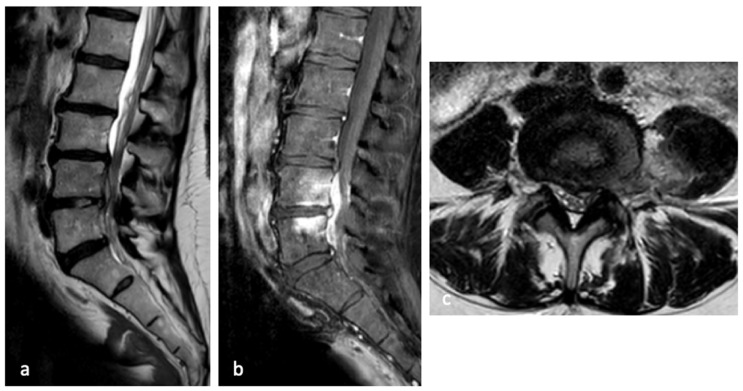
(a) T2-weighted sagittal images and (b) STIR showing hyperintensity of the L3 and L4 vertebral bodies and the presence of an intracanal abscess with retropulsion of the posterior longitudinal ligament (PLL), without evidence of large disc hyperintensity, as also demonstrated by the axial view (c).
